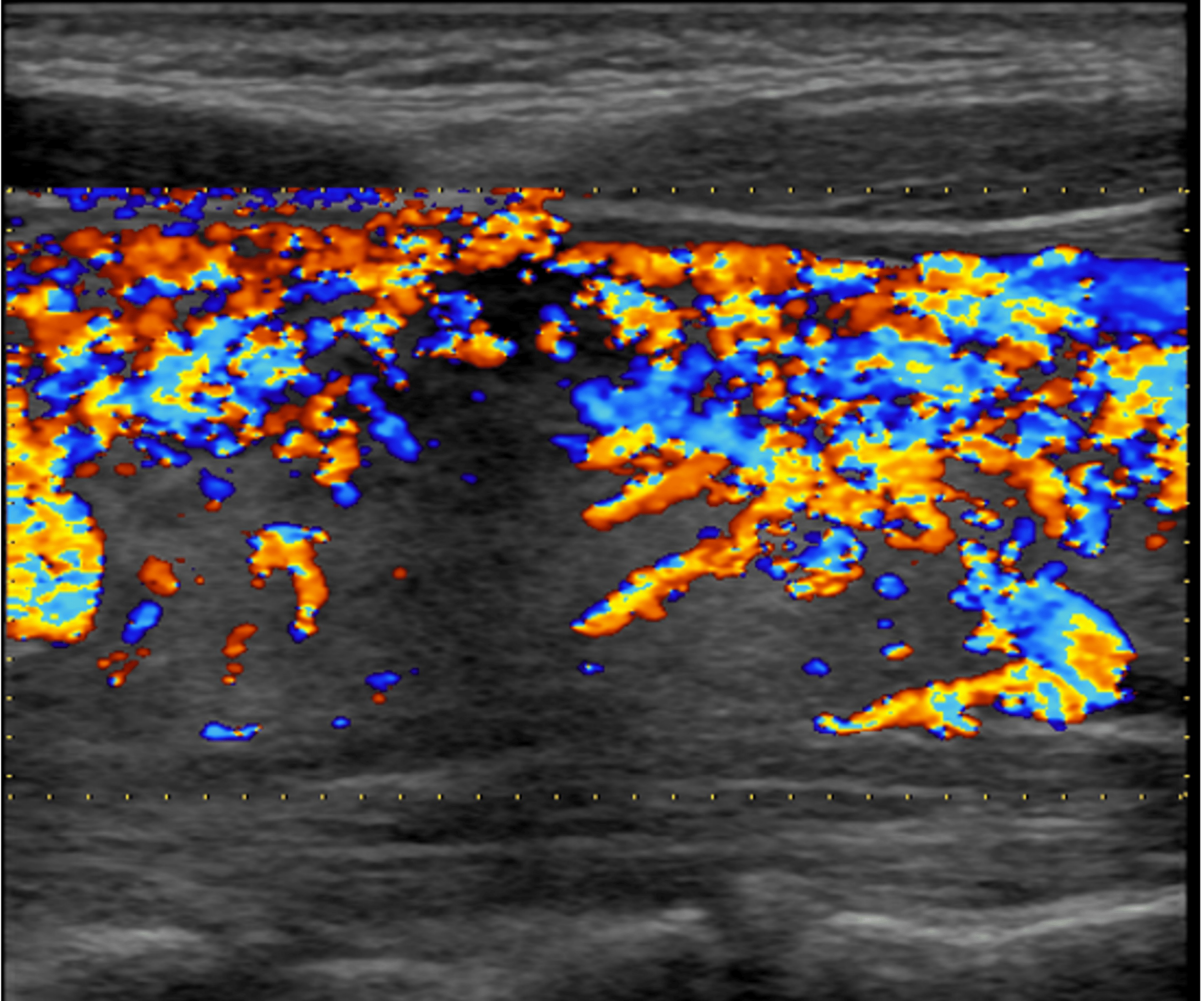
The features of the right thyroid lobe on color Doppler mode. The flow of the right lobe increased, as also shown in the left lobe.
